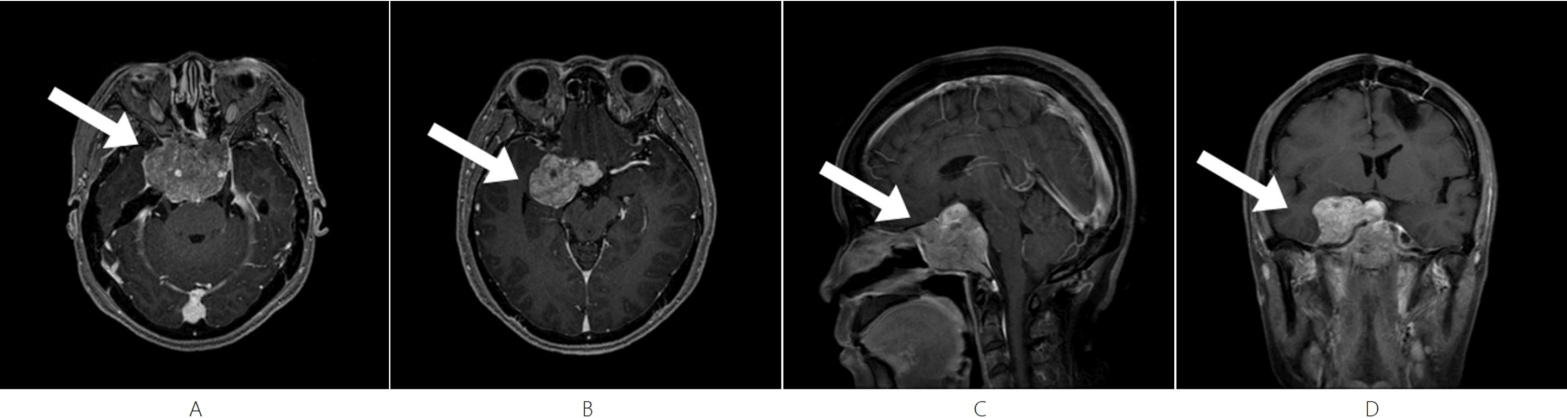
Immediately Post-IMPT - November 2023 The volume of the sellar mass is slightly reduced compared to the pre-treatment scan. (A, B) Axial T1-weighted post-contrast images show a sellar mass with heterogeneous enhancement (arrow). (C) Sagittal T1-weighted post-contrast image shows the lesion with heterogeneous mild-to-moderate enhancement (arrow). (D) Coronal T1-weighted post-contrast image shows the lesion with heterogeneous mild-to-moderate enhancement (arrow). IMPT, intensity-modulated proton therapy
